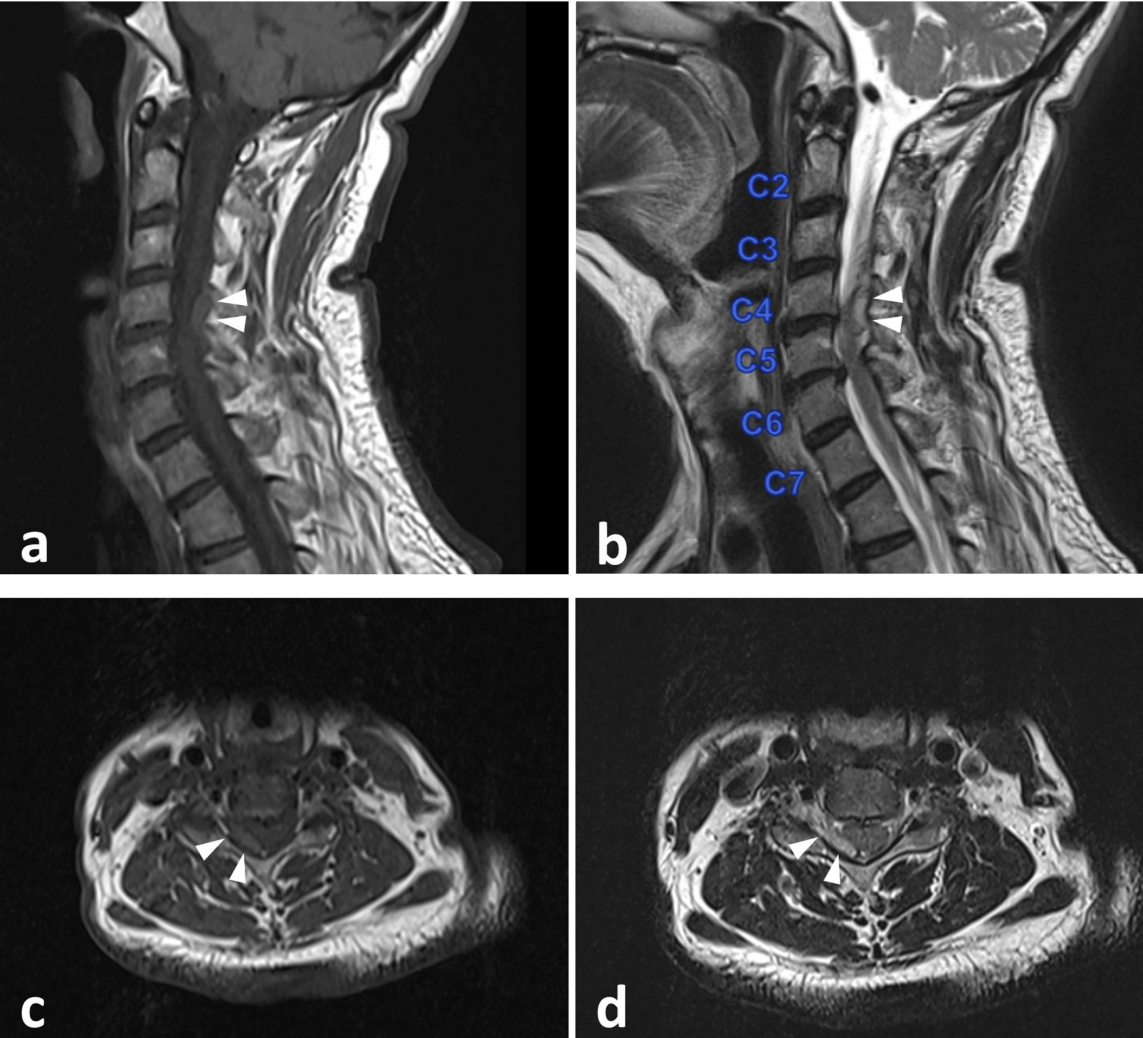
MRI of the cervical spine on day 2 showed a right posterolateral non-enhancing epidural collection spanning C3-4 to C5-6 levels, measuring 1.4 x 0.4 x 3.4 cm, which was hyperintense on the T1-weighted sequence, suggestive of hematoma (white arrows). (a) Sagittal T1-weighted sequence. (b) Sagittal T2-weighted sequence. (c) Axial T1-weighted sequence. (d) Axial T2-weighted sequence MRI: magnetic resonance imaging
